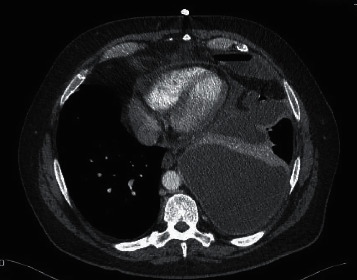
CT on initial presentation.
